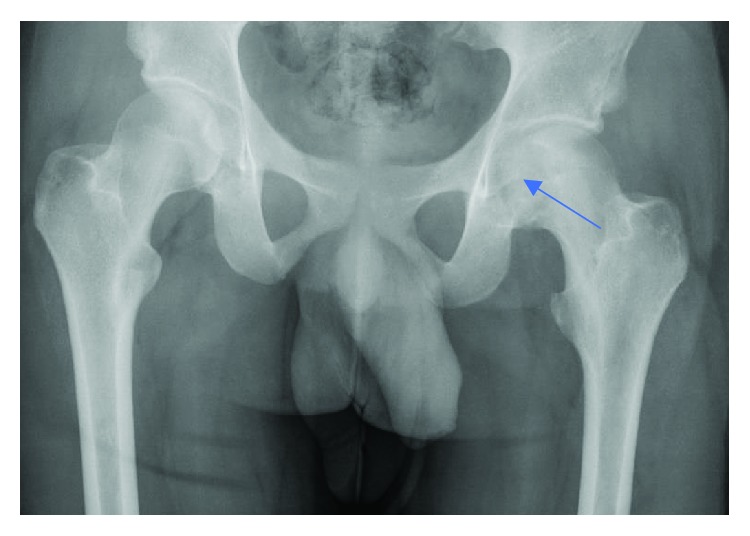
Preoperative AP pelvis X-ray showing femoral head osteolytic lesion (blue arrow).
